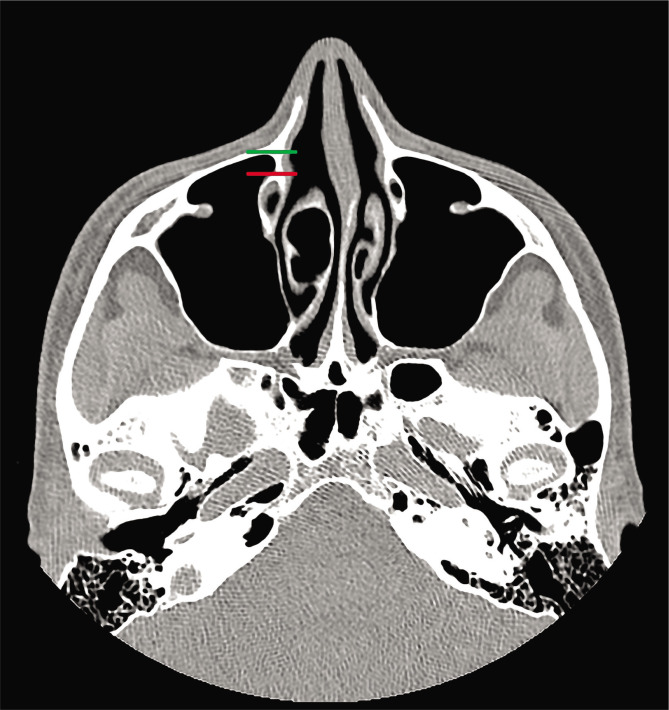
Transverse plane CT scan showing the lines used for measurements between the anterior maxillary wall (green line) and anterior margin of the nasolacrimal duct (red line).
